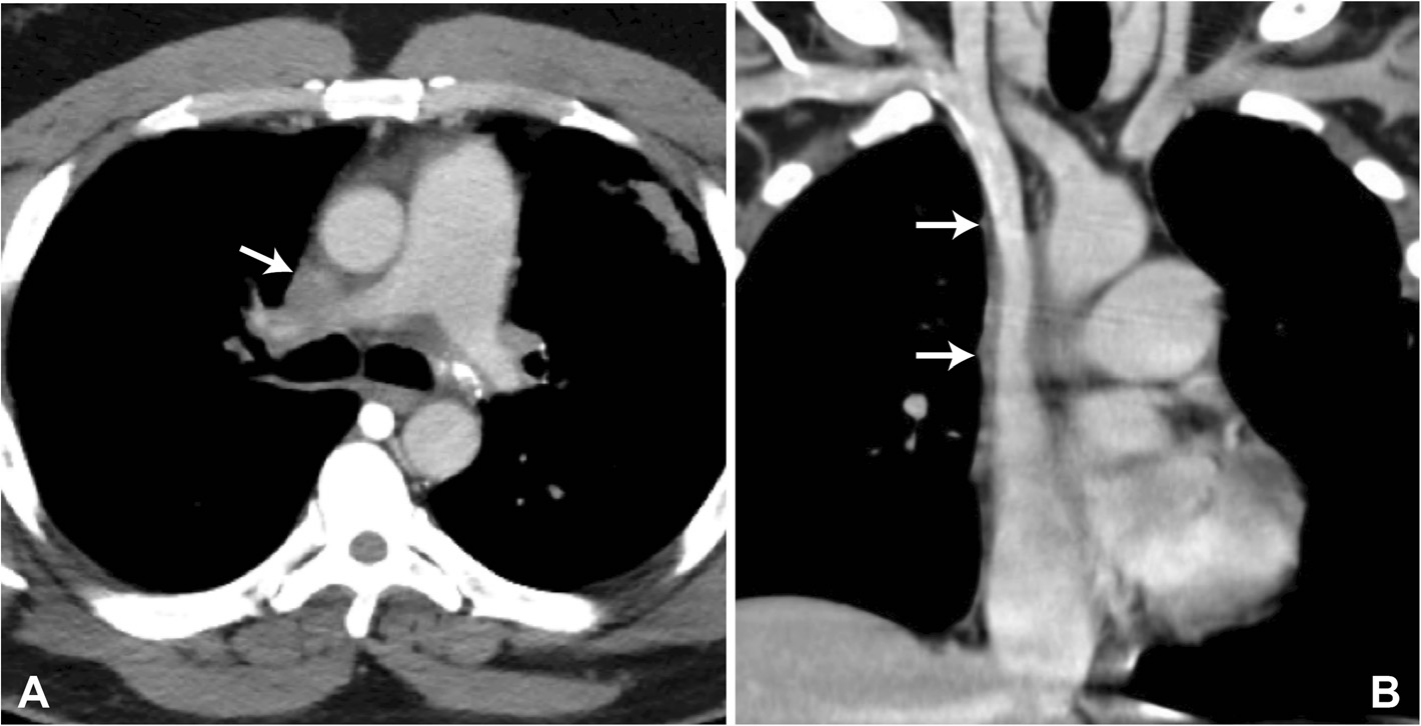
Pre and postoperative CT images. A. Preoperative axial computed tomogram with intravenous contrast demonstrating near-total obstruction of the SVC at the level of the right pulmonary artery (arrow). B. Postoperative coronal computed tomogram with intravenous contrast demonstrating wide patency of the cryopreserved thoracic aortic allograft-reconstructed SVC (arrows on proximal and distal anastomoses).
